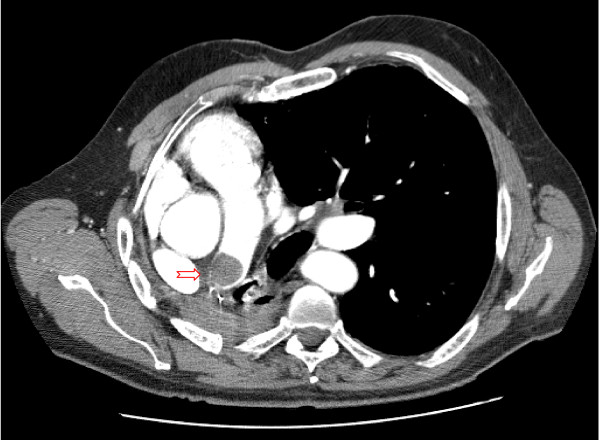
Contrasted computed tomographic (CT) scan of the chest displaying a convex-shaped filling defect in the right pulmonary artery stump.
